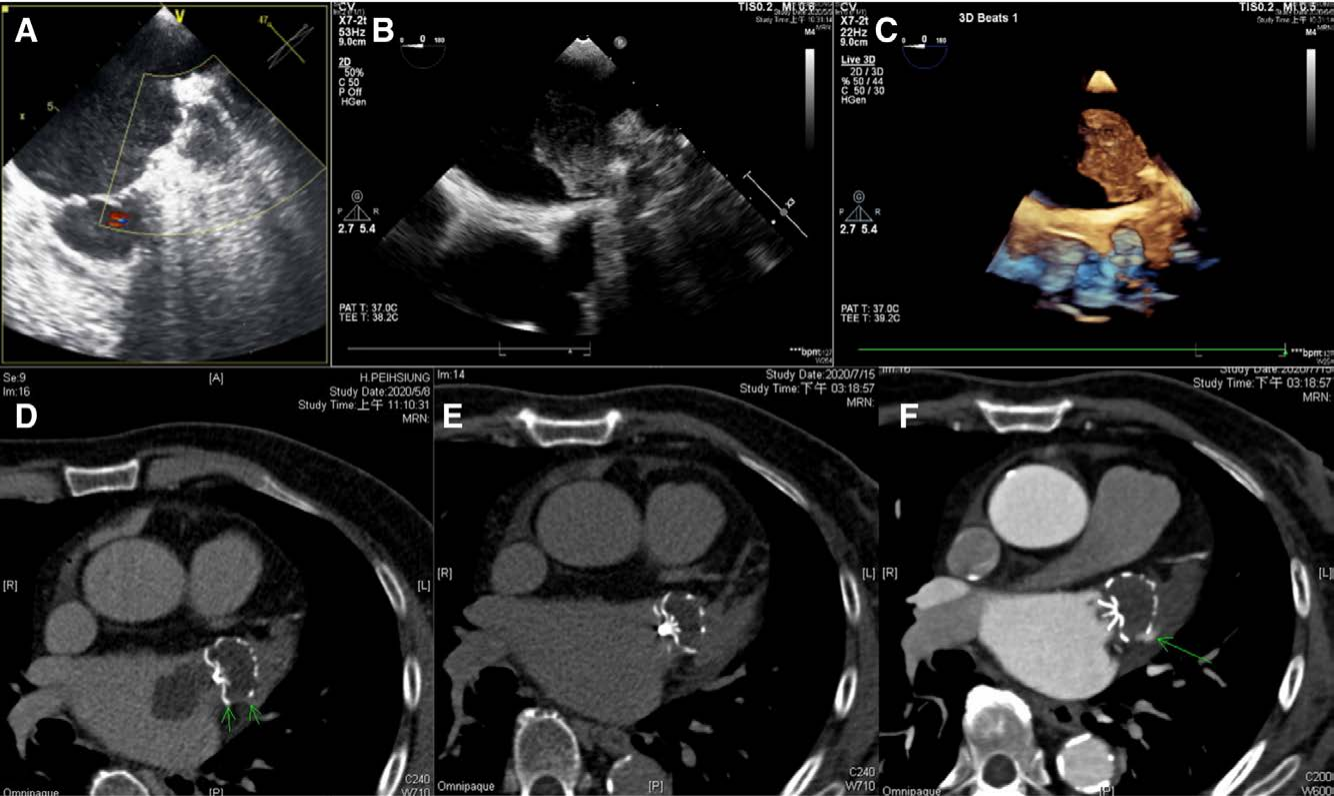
Imaging of left atrial appendage occlusion follow-up. A: TEE 45 days after left atrial appendage occlusion demonstrating a clear Watchman device surface without thrombus; B and C: Two-dimensional and three-dimensional TEE 10 months later showed a large immobile thrombus (2.3 × 4.5 cm) attached to the device surface, remarkable spontaneous echo contrast, and dilated left atrium; D: Cardiac CT angiography confirmed thrombus (3.8 × 2.4 cm) and a minimal peri-device leak; E and F Non-contrast and contrast-enhanced cardiac CT angiography after 2 months of anticoagulation therapy demonstrated complete thrombus resolution and stable minimal leakage. CT = computed tomography, TEE = transesophageal echocardiography.
